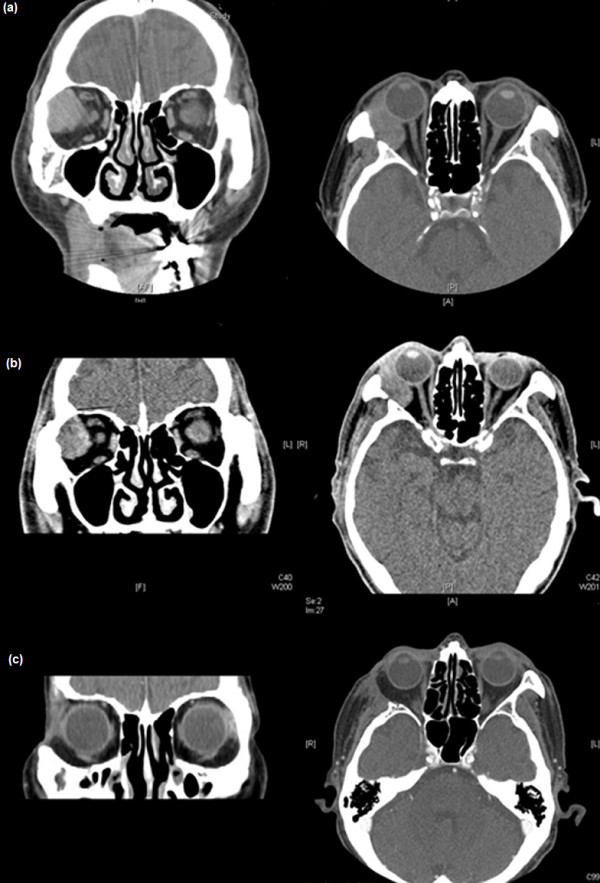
Patient 4 (75-year-old man). (a) Preoperative computed tomography (CT) scan. (b) CT scan obtained after neoadjuvant intra-arterial chemotherapy (NAIC). Note the reduction in tumour size after NAIC. (c) CT scan obtained after tumour resection with adjacent bone removal.
